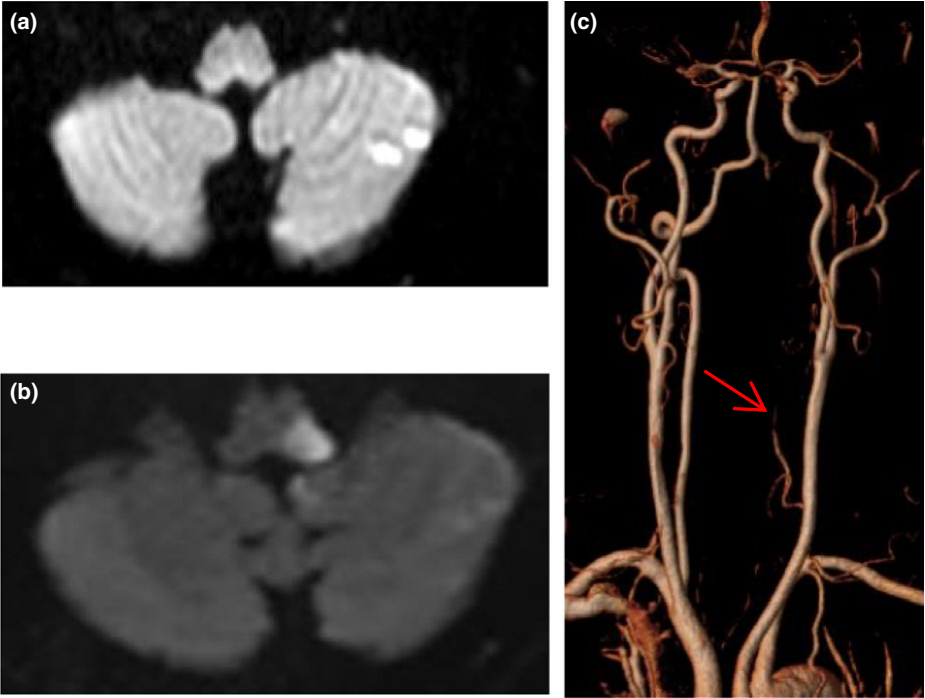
DWI image of a 61‐year old man with transient isolated vertigo showed left cerebellar infarction (a), his symptoms deteriorated 20h after onset and repeat MRI showed medullary infarction (b), the left vertebral artery was narrow and segmental absent (red arrow, c)
